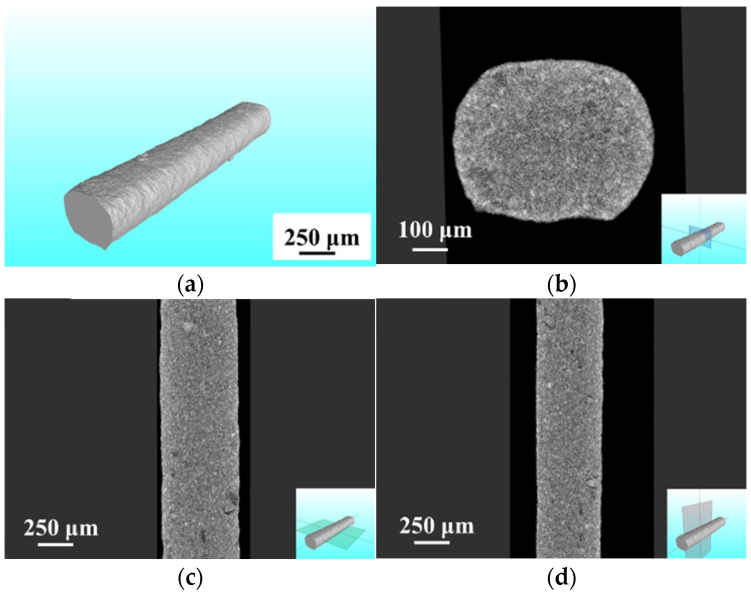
Overall morphology of the conductive circuit microstructure (CT): (a) 3D morphology; (b) cross-section morphology; (c) width direction morphology; (d) height direction morphology.
